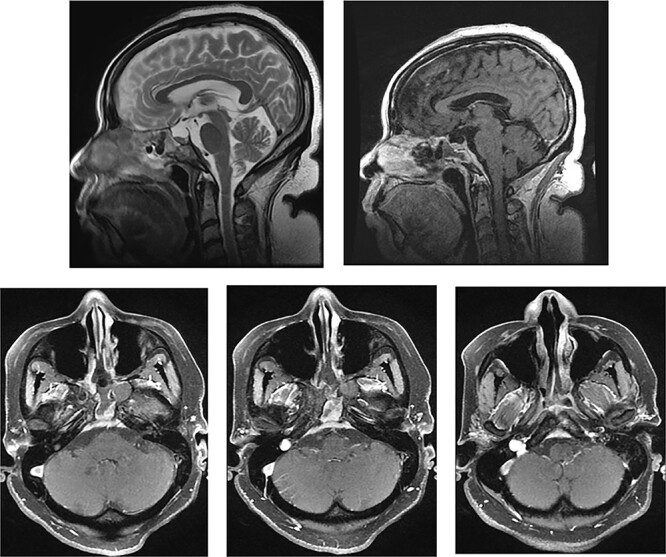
MRI of the head and orbits post-operatively (top row: sagittal T2WI and sagittal T1WI post-contrast; bottom row: axial T1WI post-contrast [from cranial to caudal]) showing fluid-filled sphenoidal sinuses, mucosal thickening and enhancement in the mucocele cavity, as well as persistent retroclival dural thickening.
